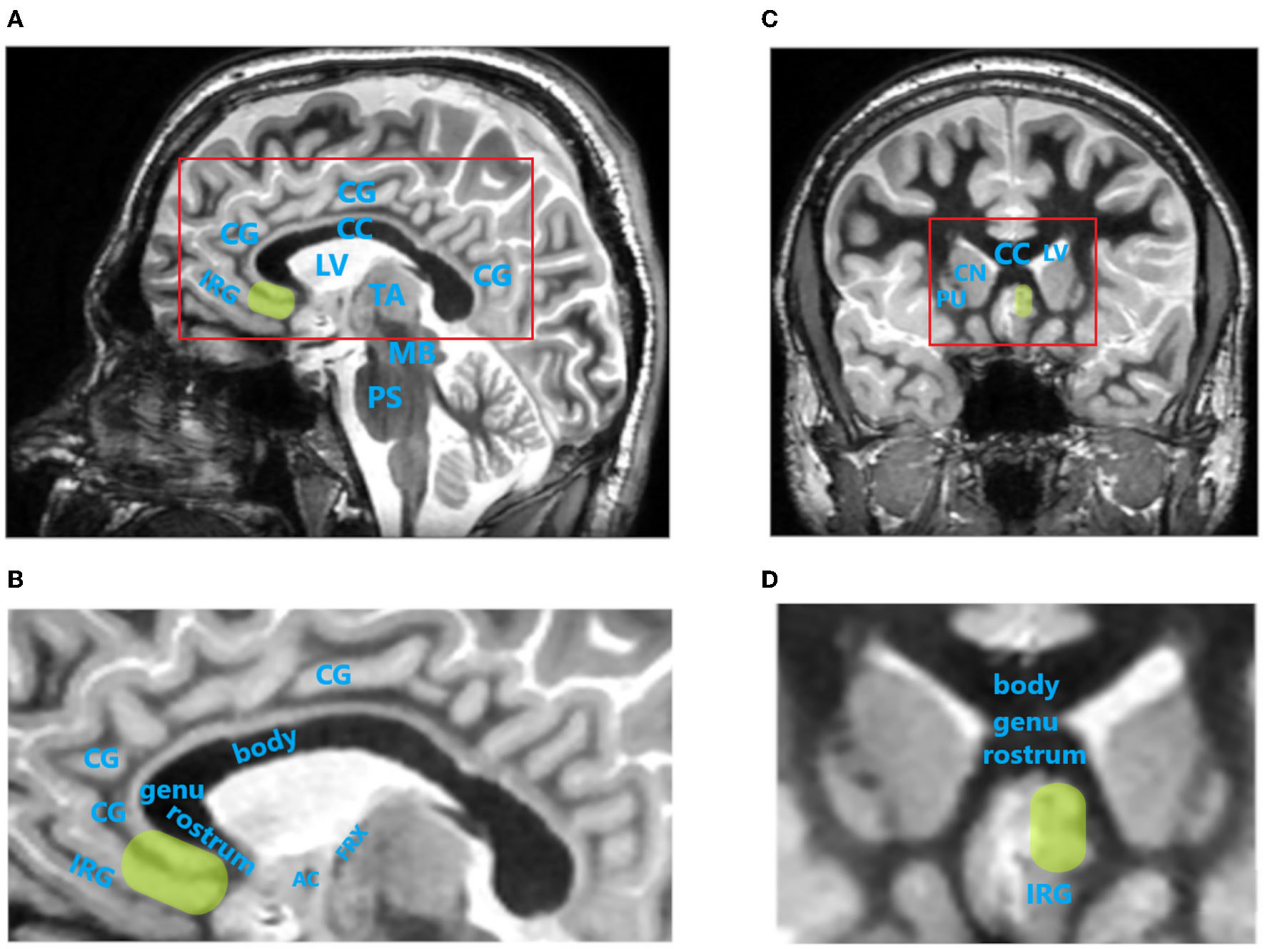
Localization of the subcallosal cingulate cortex (SCC) by magnetic resonance imaging (MRI) using Fast Gray Matter Acquisition T1 Inversion Recovery (FGATIR) sequence. SCC is indicated by green oval region. (A), Paracentral image of sagittal brain MRI scan. Red rectangle indicates brain region magnified in (B). (B), Magnified sagittal MR image of cingulate cortex region. (C), Image of coronal brain MRI scan at the level of SCC. (D), Magnified coronal MR image of cingulate cortex region. AC, anterior commissure; body, body of corpus callosum; CC, corpus callosum; CG, cingulate gyrus; CN, caudate nucleus head; genu, genu of corpus callosum; FRX, ascending column of fornix; IRG, inferior rostral gyrus; LV, lateral ventricle; MB, midbrain; PS, pons; PU, putamen; rostrum, rostrum of corpus callosum; TA, thalamus. MRI images were acquired on 3T SIGNA Architect scanner (GE Healthcare).
